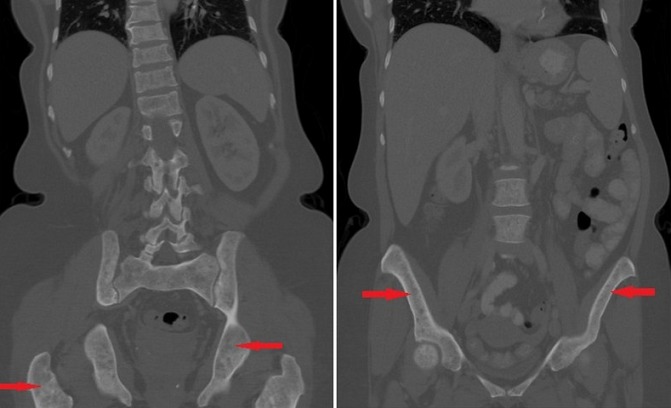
computed Tomography (CT) of the abdomen and pelvis showing osteosclerosis of pelvic bones
